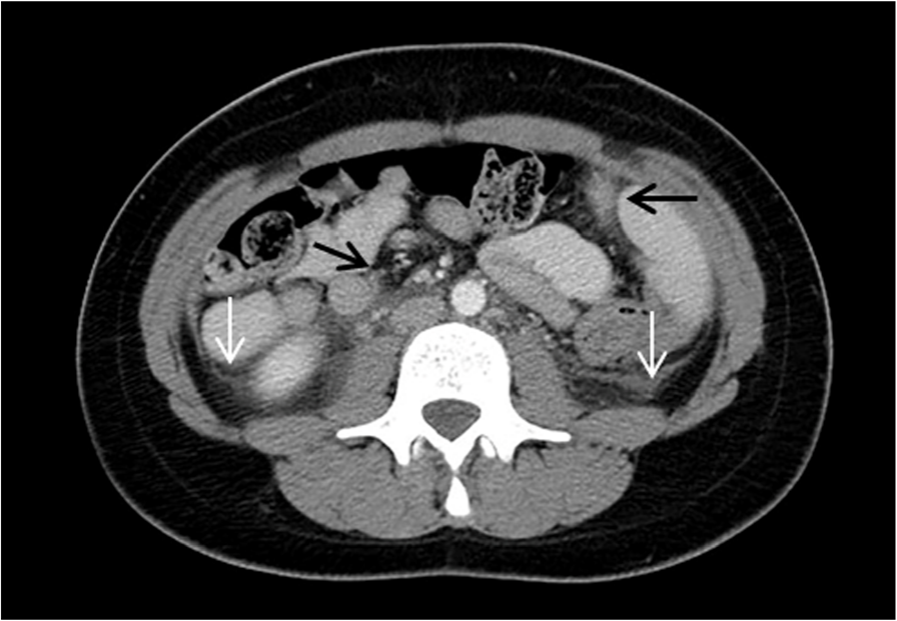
Familial Mediterranean fever. Selected axial CT image of the abdomen after oral and intravenous contrast administration in a 14-year-old girl presenting to the Emergency department with acute abdominal pain showing small ascites (white arrows) and diffuse intra-abdominal fat stranding (black arrows). Solid organs, bowel, and appendix were normal (not shown). Findings represent peritonitis without detectable underlying cause
